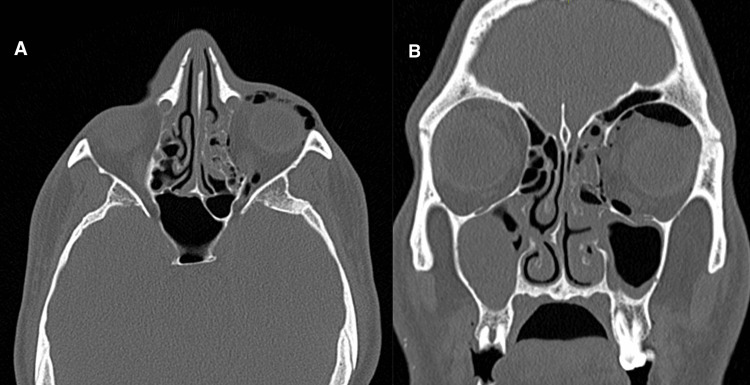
Coronal and axial views of the CT bone window Axial (A) and coronal (B) CT acquisitions with bone algorithms, demonstrating a blow-out fracture of the left orbital medial wall and left orbital floor.
